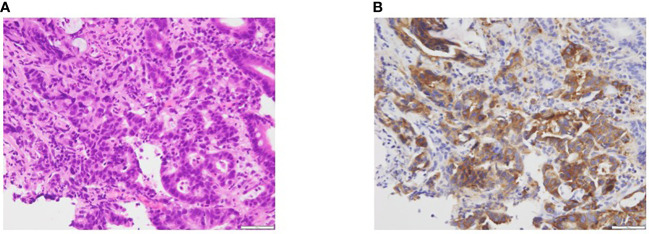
(A) Course of tumor markers (carcinoembryonic antigen [CEA] and carbohydrate antigen 19-9 [CA 19-9]) while receiving treatment with FOLFOX and alectinib. (B) Abdominal computed tomography showing multiple liver metastases pre-FOLFOX therapy, increased tumor volume 4 weeks after the FOLFOX therapy, and reduction of liver metastases 6 weeks after alectinib. the second follow-up CT imaging showed continuing response for liver metastases.
